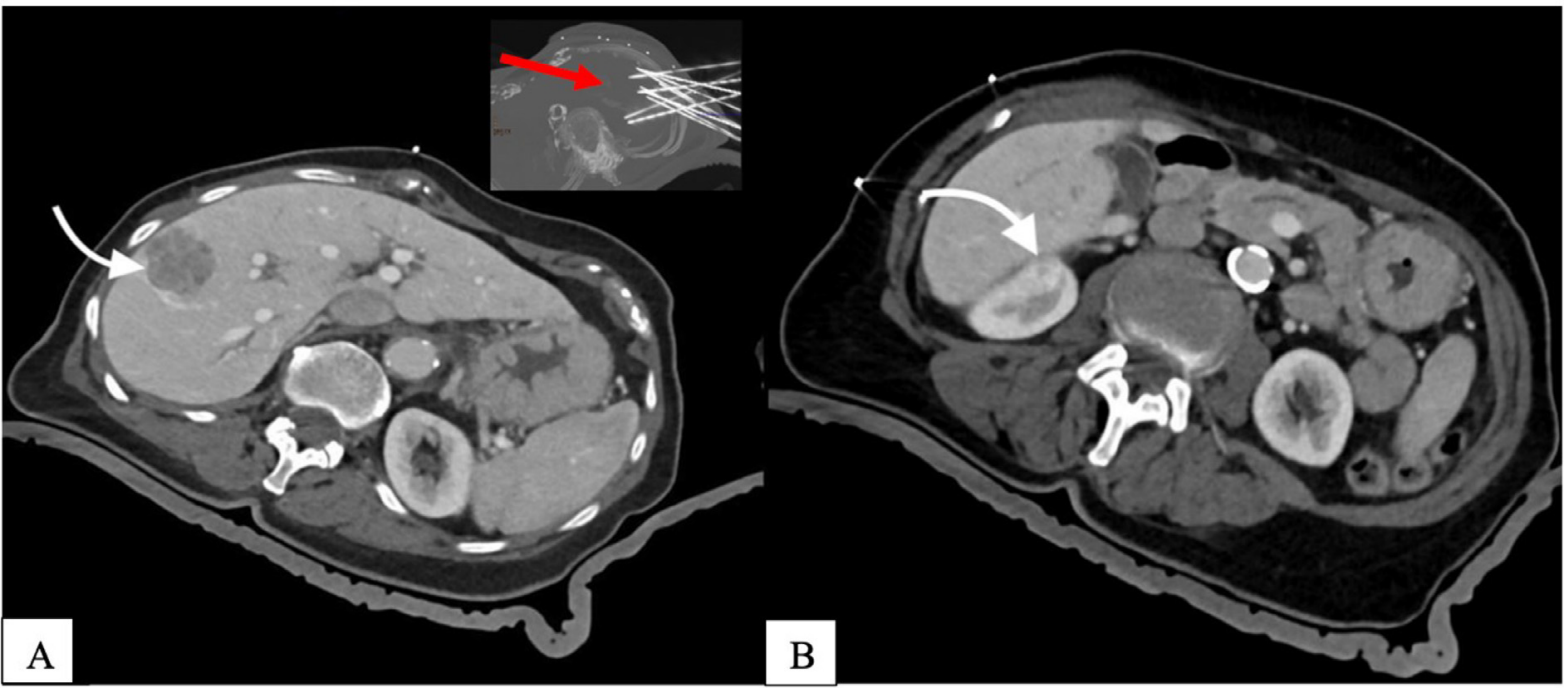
(A-B): Planning CT scan in arterial phase of the SRFA. (Fig. 3 A-B left and right axial view): Planning CT scan in arterial phase of the SRFA procedure with a 2,4 cm mass in liver segment VIIII and a 1, 7 cm mass on the superior pole of the right kidney. Maximum Intensity Projection (MIP) of the nonenhanced control CT scan showing the position of 9 coaxial needles and the position of 2 coaxial needles in the right kidney (right upper corner Fig. 3A).
